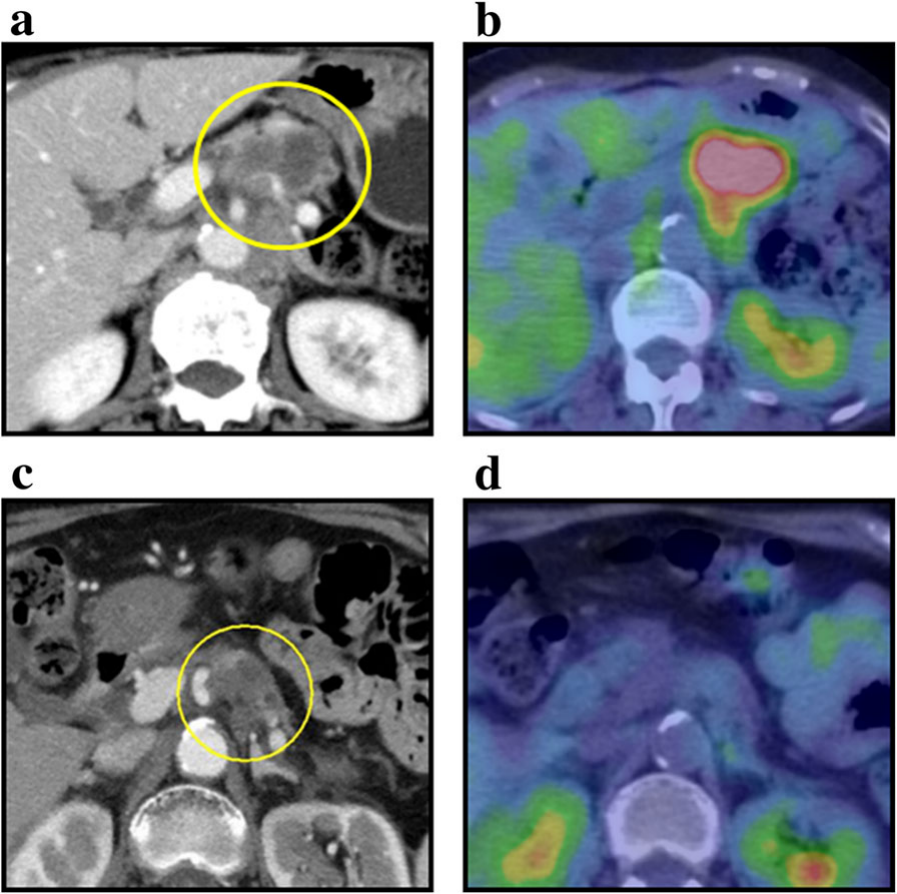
An enhanced CT revealed a 45-mm tumor in the body of pancreas (a). The SUV (max) of the tumor was 6.2 in a PET-CT (b). After the chemoradiation for 15 months, the size of the primary tumor was reduced to 25 mm (c). The abnormal uptake in a PET-CT disappeared (d)
